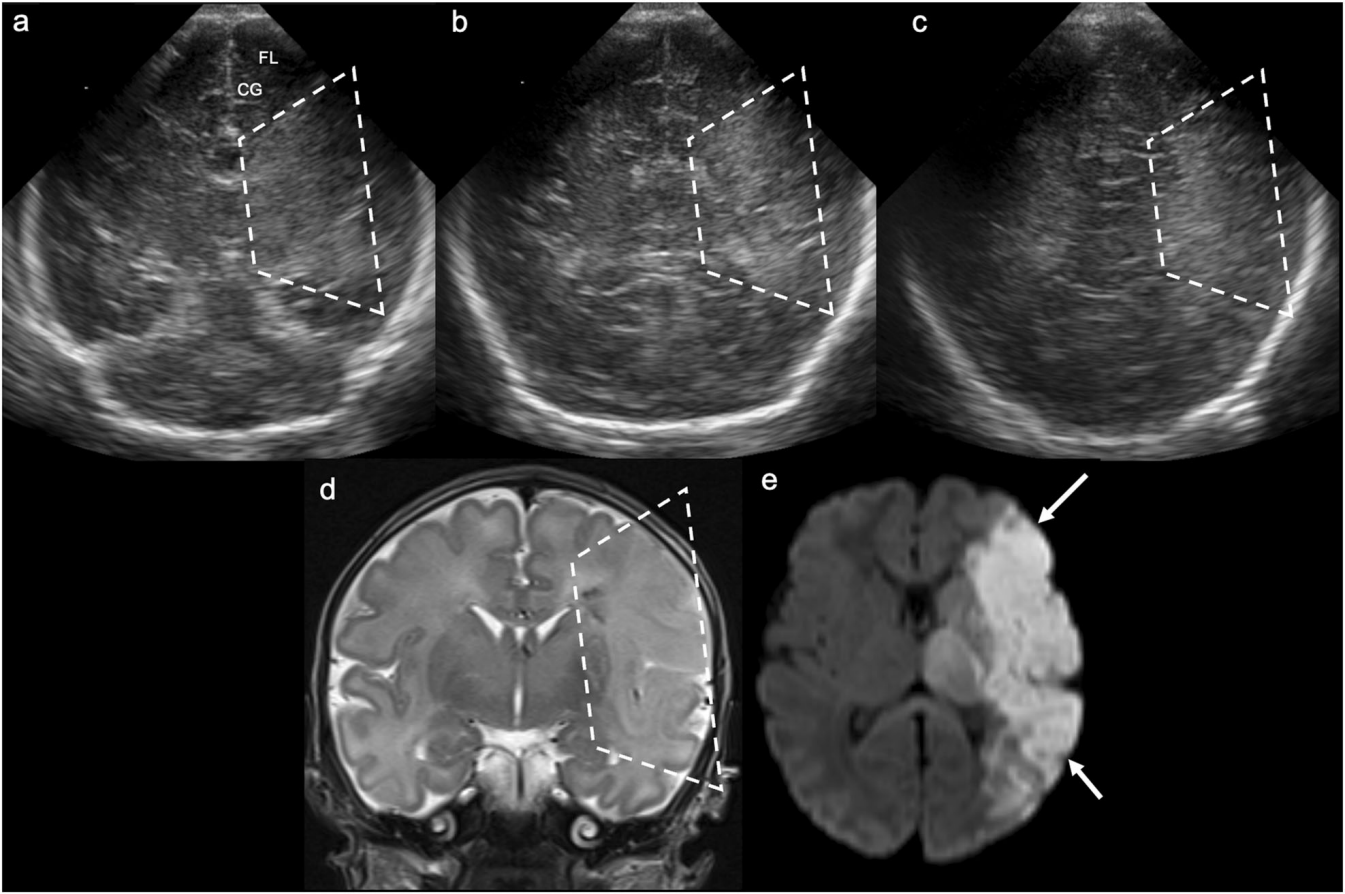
Perinatal arterial ischaemic stroke. Day 2 ultrasound in a 36 + 1-week gestation neonate with seizures. a–c Coronal images at the level of the third ventricle (a), lateral ventricular trigones (b), and occipital lobes (c) demonstrate ill-defined, wedge-shaped increased echogenicity in the left cerebral hemisphere (dashed lines), sparing the medial left frontal lobe (FL) and cingulate gyrus (CG); there is associated brain swelling with loss of sulcation. d Coronal T2WI demonstrates corresponding hyperintensity in the left cerebral hemisphere, involving both cortex and subcortical white matter, with loss of normal grey-white matter differentiation (dashed lines). e Axial trace DWI demonstrates restricted diffusion (ADC map not shown) in the left cerebral hemisphere corresponding to the left middle cerebral artery territory (solid arrows)
